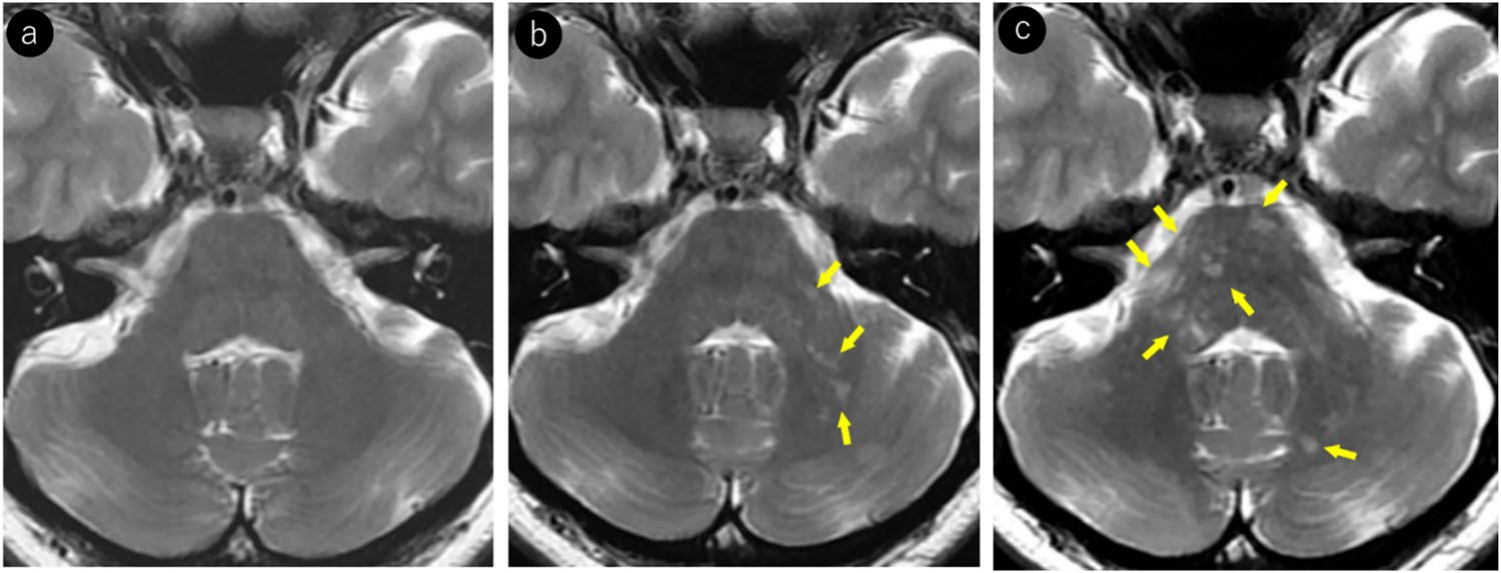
Woman in her 40 s, who had been undergoing fingolimod treatment for MS for 8 years started experiencing walking difficulties a few weeks prior to presentation. a MRI performed 3.5 years prior showing no abnormalities in the infratentorial region. b T2-weighted imaging 2 years prior demonstrating subtle punctate and small hyperintense lesions around the left dentate nucleus and left middle cerebellar peduncle (yellow arrows). These lesions were considered asymptomatic at the time as the patient exhibited no clinical symptoms. c At the time of symptom onset, T2-weighted imaging reveals a punctate pattern and milky way appearance in the pons, bilateral middle cerebellar peduncles (right predominant), and around the left dentate nucleus (yellow arrows). JCV was detected in cerebrospinal fluid examination and PML was diagnosed
